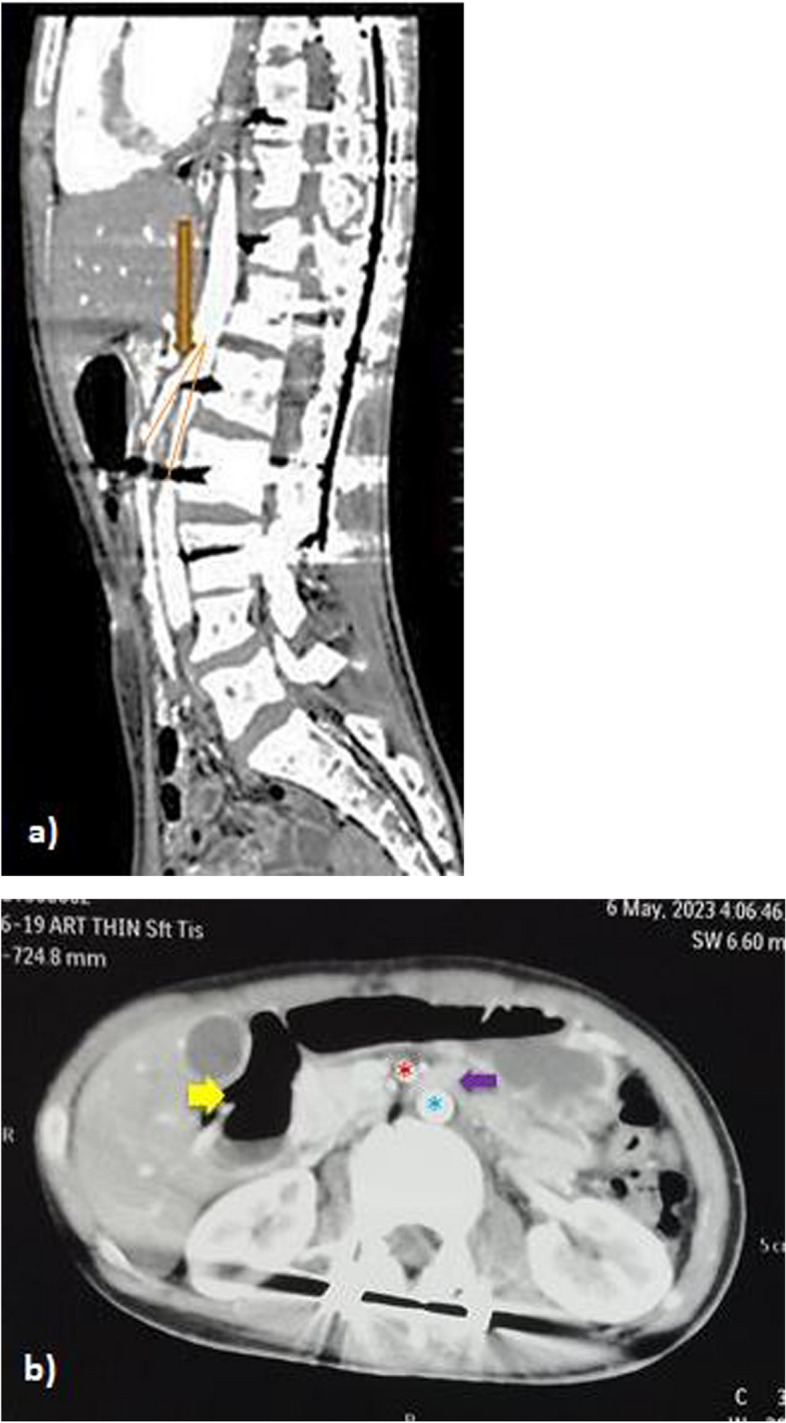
CT angiogram showing reduction in aorto-mesenteric distance and aorto-mesenteric angle with dilated proximal duodenum and collapsed duodenum distal to the compression site between the aorta and SMA. a Arrow points to the angle formed between the abdominal aorta and its branch, the Superior Mesenteric artery. It is reduced to 20 degrees (normal – 38–65 degrees). This causes an extrinsic vascular compression over the third part of duodenum causing symptoms of intestinal obstruction. b Yellow arrow points to the dilated proximal duodenum, Red asterisk is the SMA, Green asterisk is the abdominal aorta, Purple arrow is the collapsed distal duodenum. Aorto-mesenteric distance is 4.4 mm (normal 10-28 mm)
